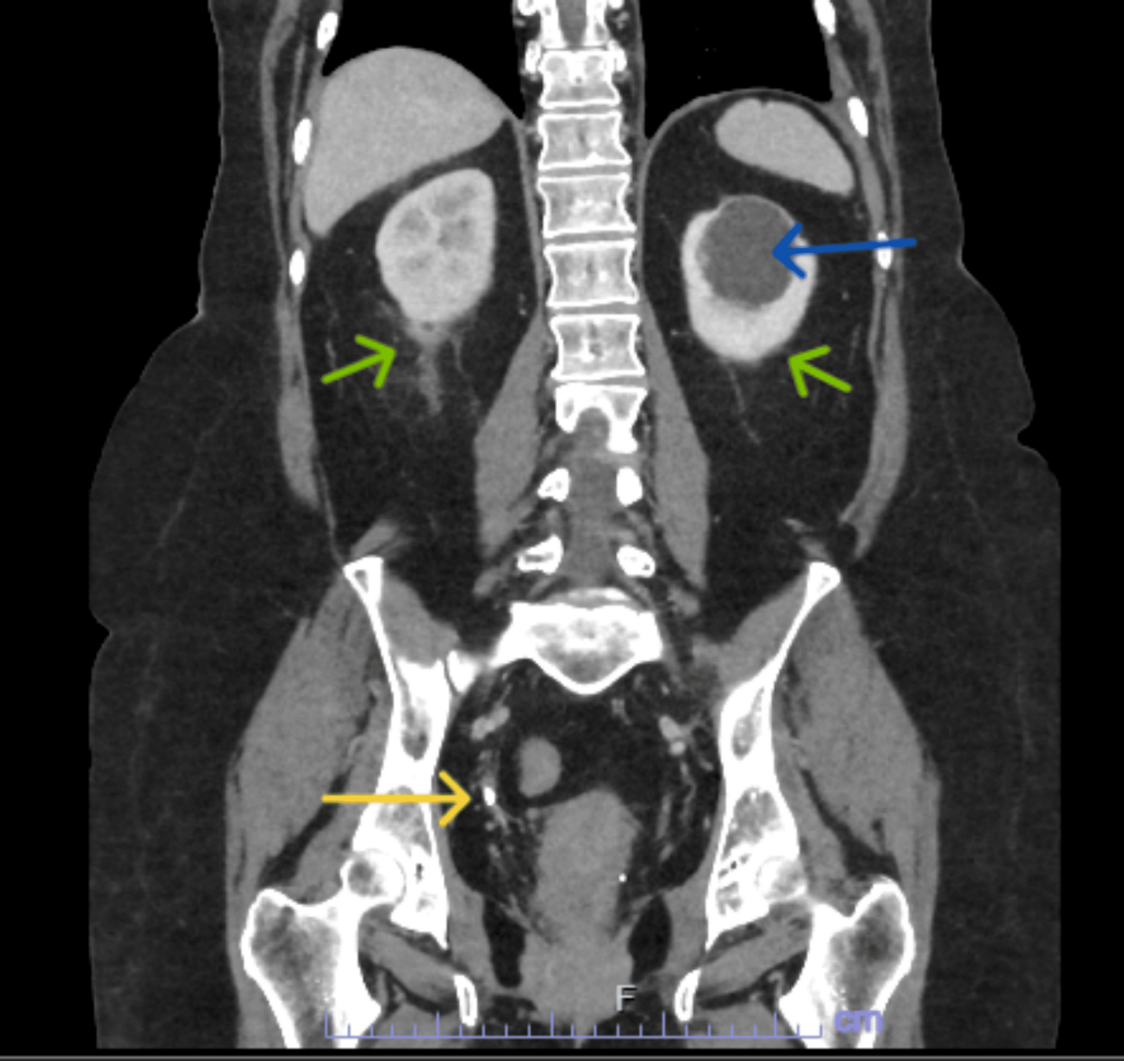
Coronal view of CT abdomen and pelvis showing a distal right 6 mm ureteric stone (yellow arrow). Also note bilateral perinephric stranding (green arrows) and a parapelvic cyst on the left (blue arrow).
